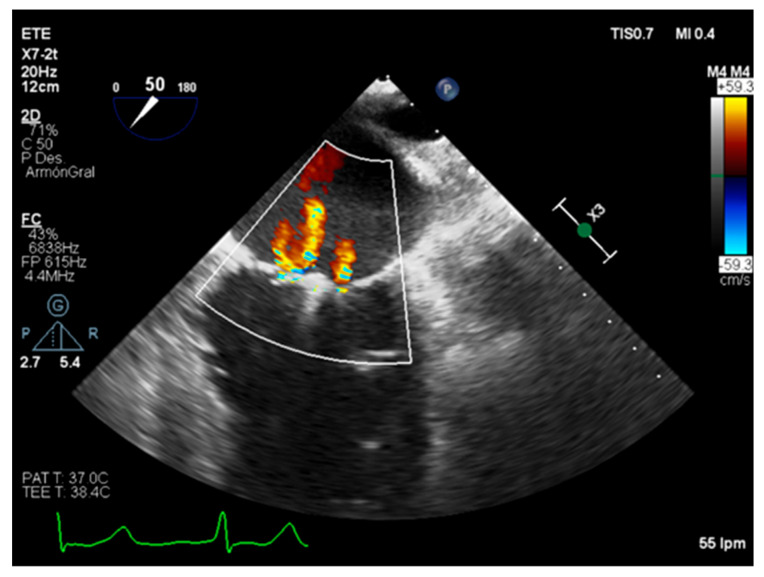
Transesophageal echocardiography. Disproportional mitral regurgitation in a non-ischemic dilated cardiomyopathy.
